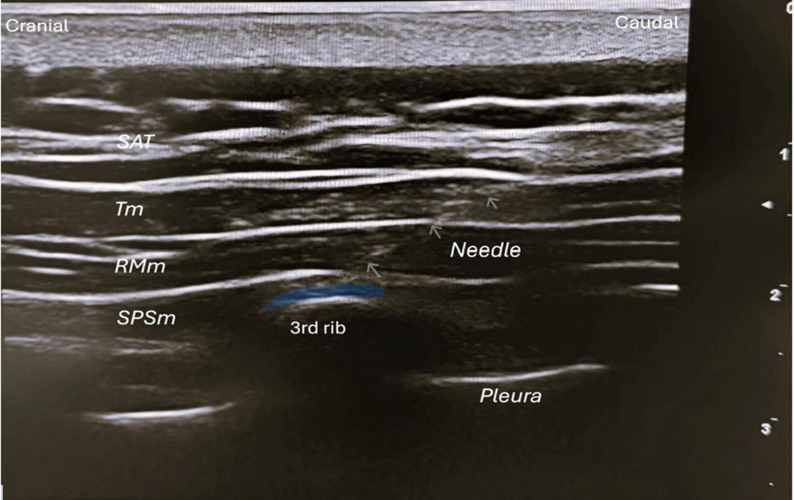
Serratus posterior superior intercostal plane block (SPSIPB). SAT: Subcutaneous adipose tissue, Tm: Trapezius muscle, RMm: Rhomboid major muscle SPSm: Serratus posterior superior muscle. The blue-shaded area indicates the intended target injection plane for the SPSIPB, with the needle advanced in a caudal-to-cranial direction under linear ultrasound probe guidance
